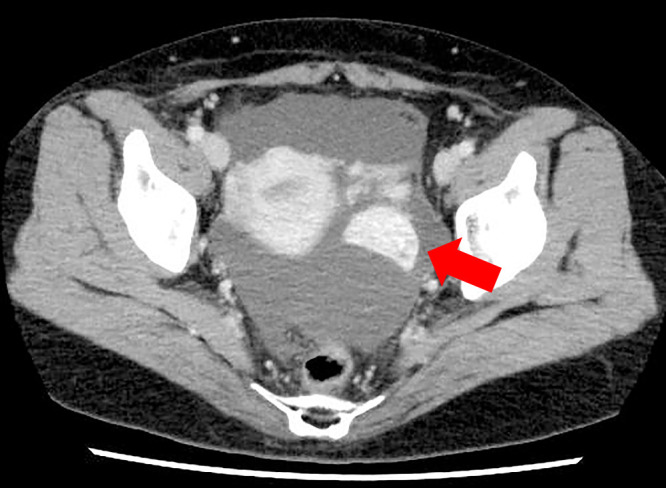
Contrast-enhanced computed tomography image of the pelvic region (Case 1) A solid tumor with strong contrast enhancement was found in the left pelvis.
